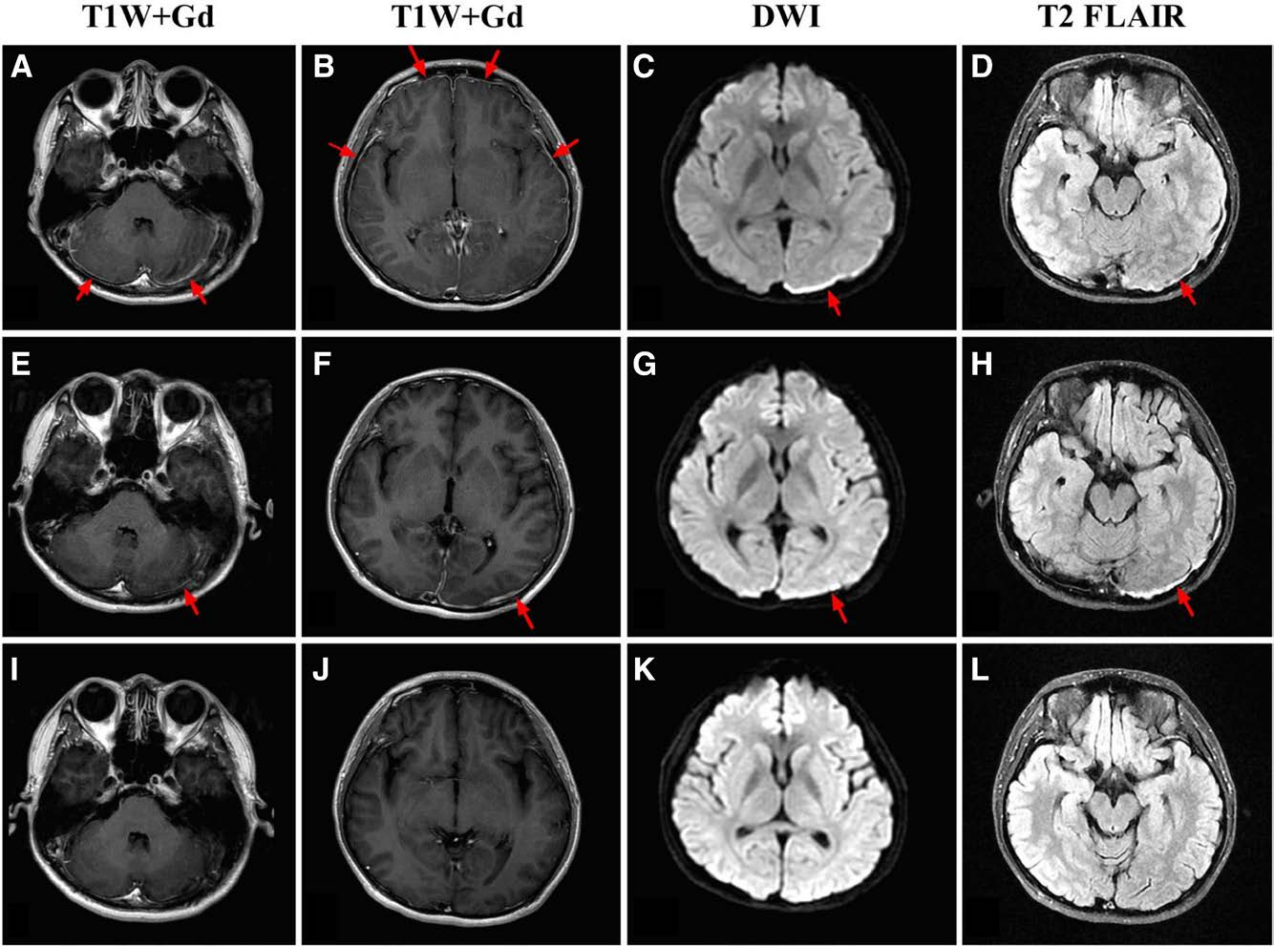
(A–D) In phase I, enhanced brain MRI revealed thickening of the bilateral dura mater, particularly on the left side. (E–H) In phase I, enhanced brain MRI revealed a slight improvement in the lesion of the dura mater during the process of immunotherapy. (I–L) In phase II, enhanced brain MRI revealed that thickening of dura mater was relieved after approximately 1 month of comprehensive treatment.
